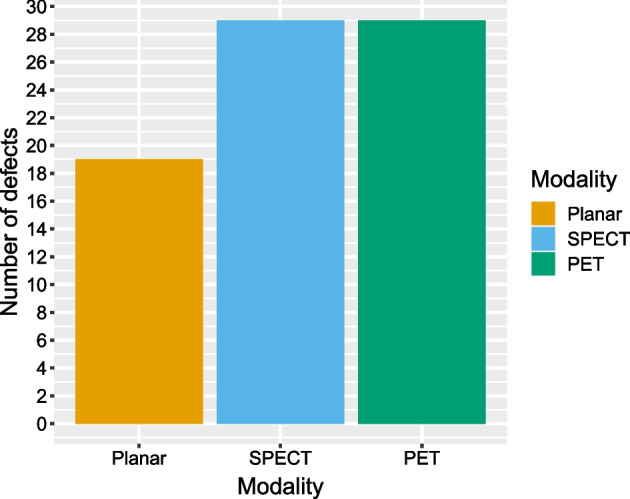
Total number of defects found per modality, final consensus interpretations. *SPECT* single photon emission computed tomography; *PET* positron emission tomography
